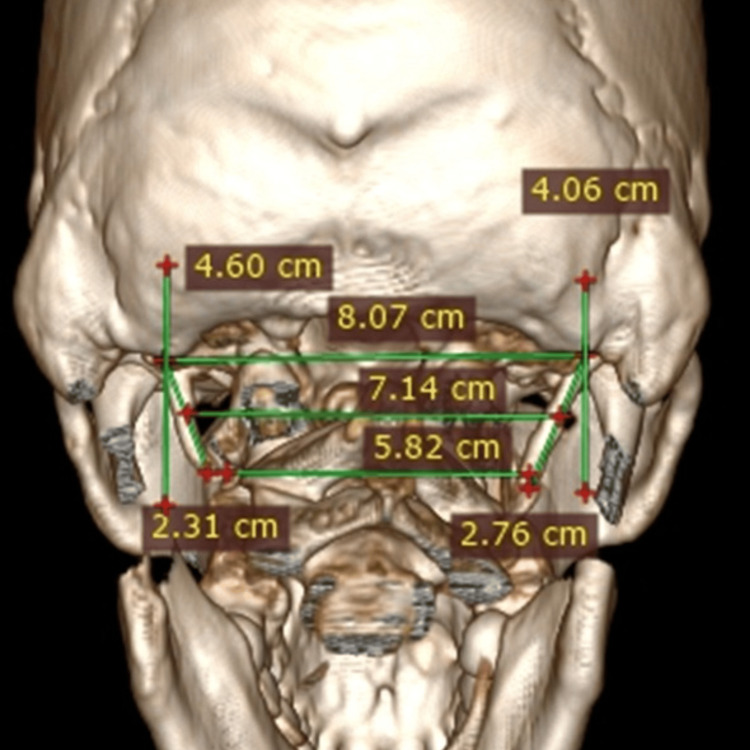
Normal length (<3 cm long) of styloid process on posterior view on 3D CT. 3D CT, three-dimensional computed tomography.
